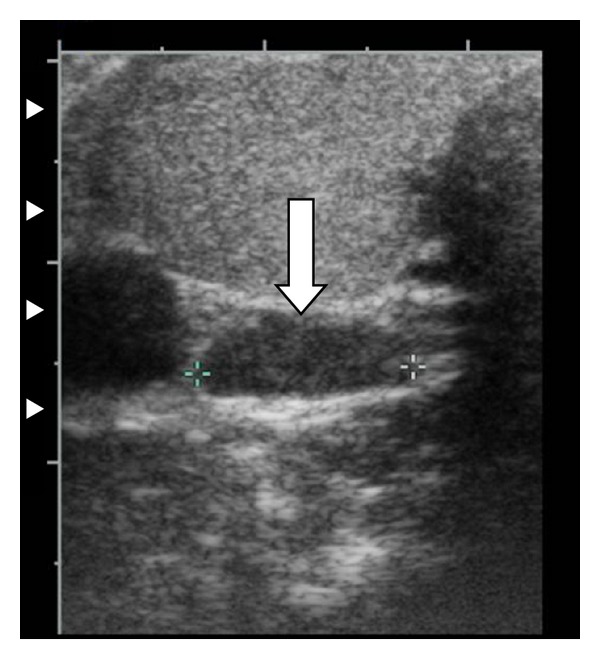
Ultrasound image demonstrates a hypoechoic mass, 1.9 × 0.4 × 1 cm, inferior and posterior to the right lobe of the thyroid, consistent an enlarged right inferior parathyroid gland.
